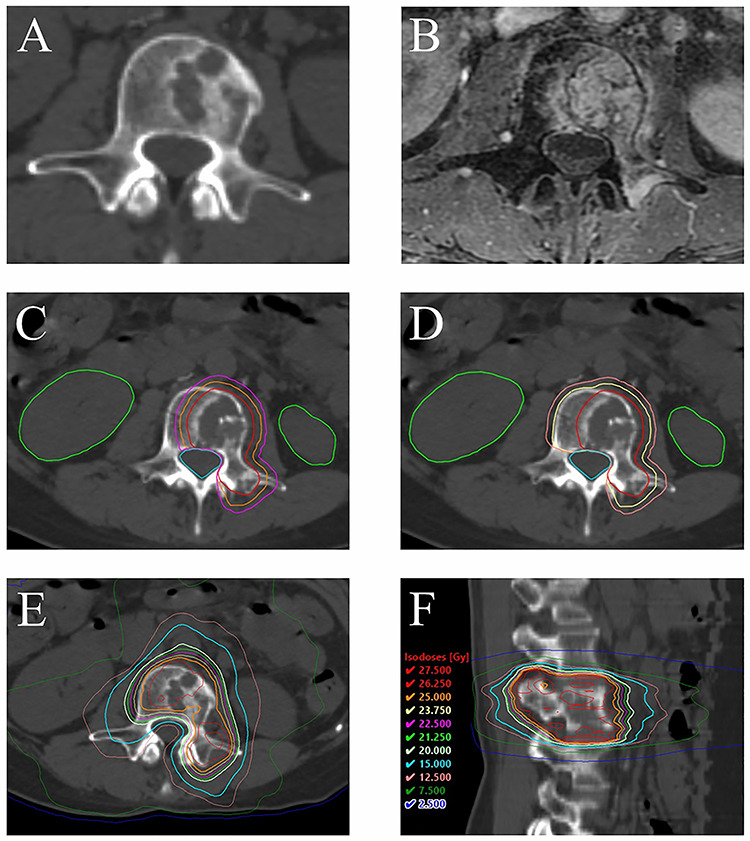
Images, contoured targets and dose distribution in one of the cases: a 46-year-old female descending colon cancer patient with spinal metastases (L3) reirradiated by SBRT. She had undergone palliative radiation therapy for the same spinal metastases at a radiation dose of 30 Gy administered in 10 fractions. The interval between the first radiation therapy and reirradiation was 9.9 months. (A) CT showing the tumor before the reirradiation. (B) MRI showing the tumor before the reirradiation (contrast-enhanced T1-weighted image with fat suppression). (C, D) Contouring (red line: GTV; orange line: CTV; purple line: PTV; yellow line: CTV_sub; light pink line: PTV_sub; green line: kidneys; cyan line: spinal cord). (E, F) dose distribution of the treatment.
